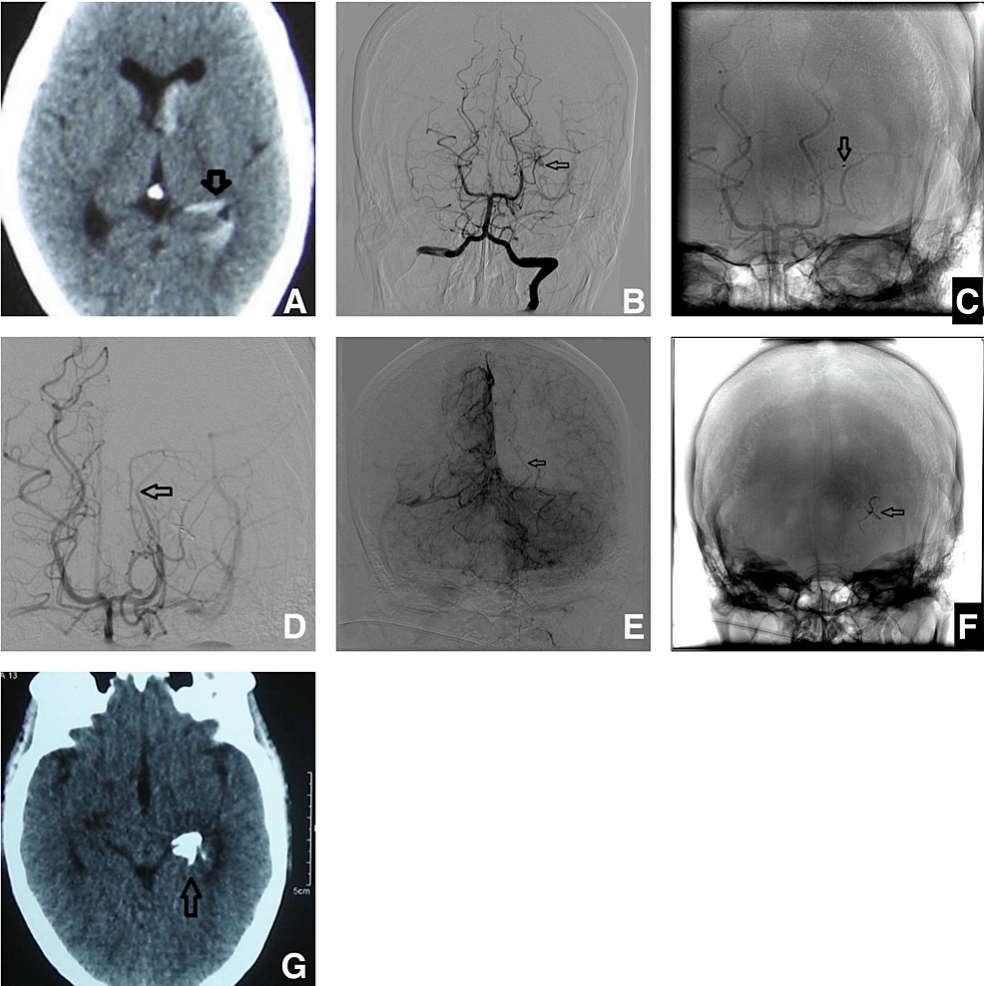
CT scan shows hemorrhage in the left choroid fissure with extension into the temporal and frontal horns of the lateral ventricle pointed by the arrow (A), DSA anteroposterior view (B) showing a small AVM nidus supplied by the posterolateral choroidal artery marked by the arrow. Fluoroscopic image (C) shows the catheter tip marked by the arrow. DSA image (D) shows complete exclusion of the AVM nidus. However, there is a non-visualization of the left distal posterior cerebral artery, which is reformed in the delayed phase (E); the arrow points to the left posterior cerebral artery, which appears to be in spasm. Fluoroscopic image (F) shows the Onyx cast marked by the arrow. Post-embolization CT shows the Onyx cast; note the streak of the cast in the choroid fissure marked by the arrow (G). A follow-up angiogram after one year showed no recurrence (not shown here). AVM - arteriovenous malformation; DSA - digital subtraction angiogram
